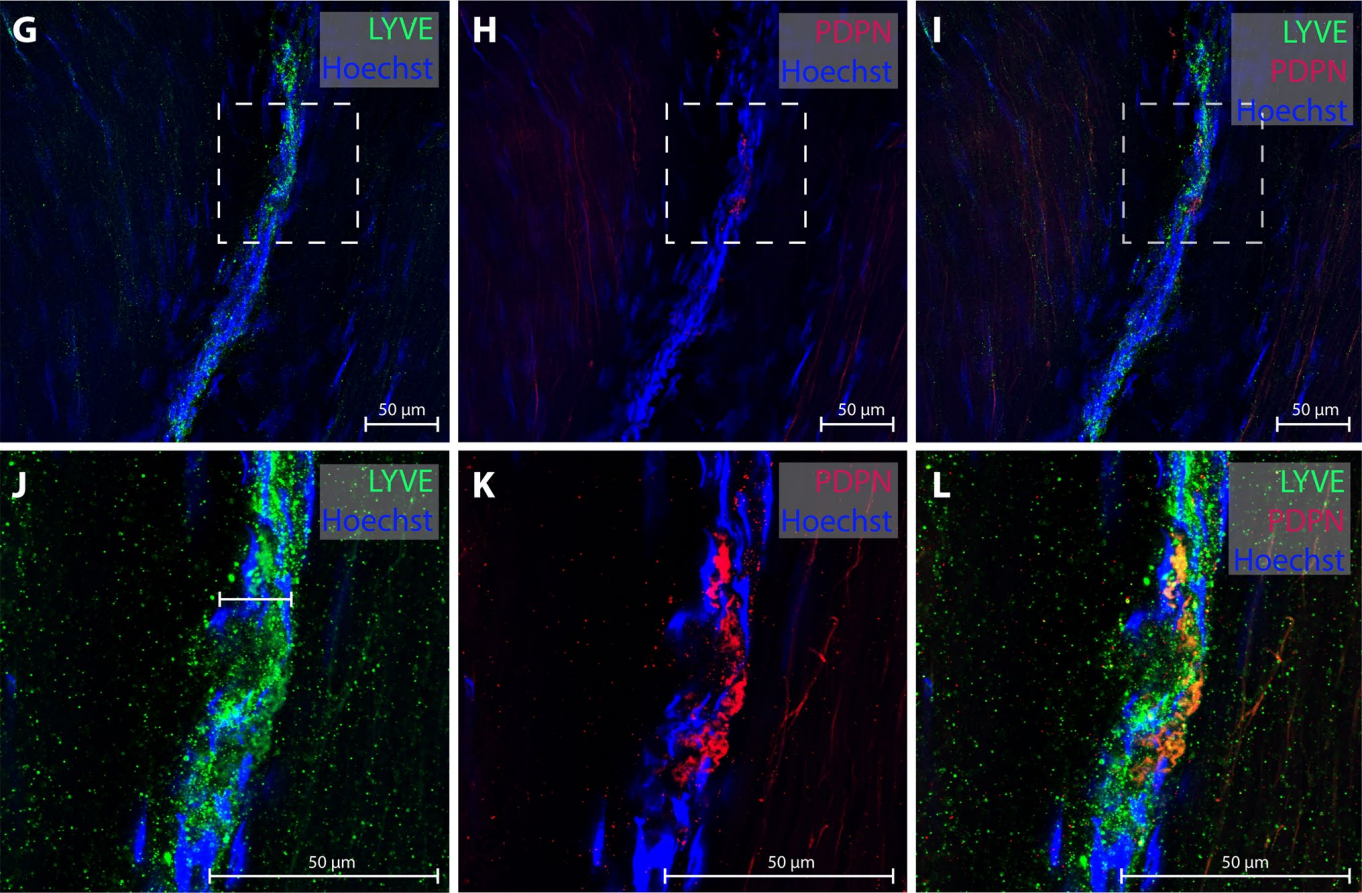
Human dural lymphatic vessel from the upper frontal convexity. Co-localization of the lymphatic markers lymphatic vessel endothelial hyaluronan receptor 1 (LYVE-1) and podoplanin (PDPN) confirms the presence of meningeal lymphatic vessels in dura mater of patients (panels J–L are magnification of panels G–I). The vessels have a diameter at micrometer scale and would not be expected to be delineated at mm-scale MRI. Courtesy of Fig. 7 G–L from Quesada et al. FBCNS) [1], reprinted under the terms of the Creative Commons Attribution License (CC BY)
